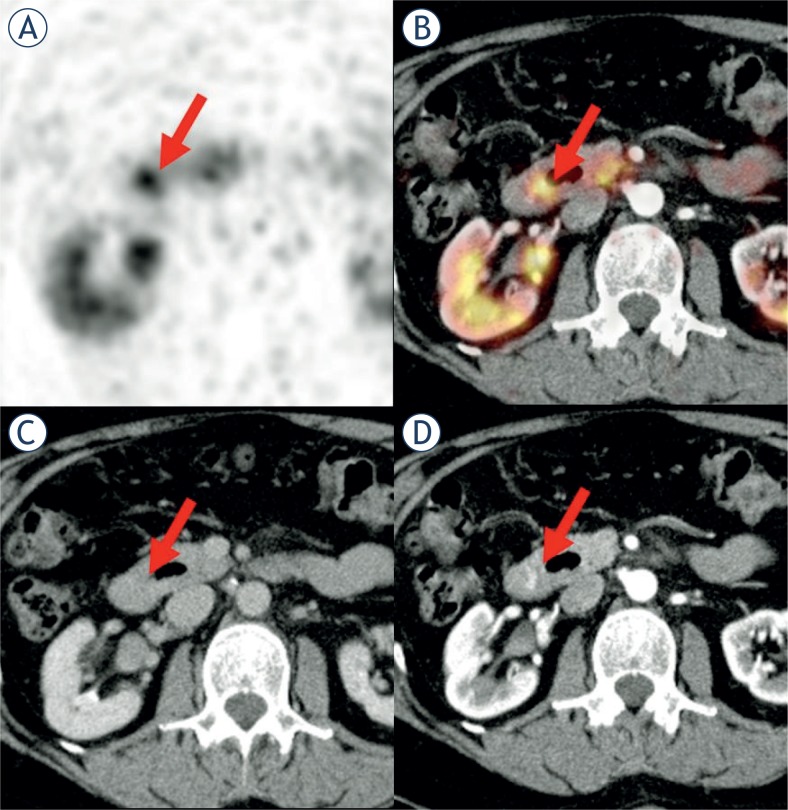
Suspicious Ga-68-DOTATOC PET lesion (#2) (A) located in the duodenum (B) without a clear correlate in the venous-phase CT (C), but arterial hyperperfusion (D).
